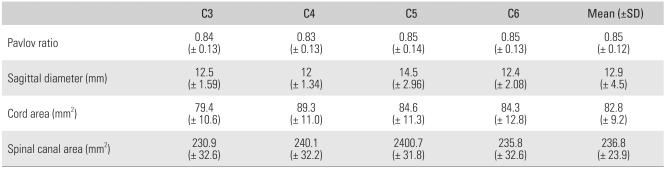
Pavlov Ratio in the Plain X-ray and Sagittal Diameter, Cord Area, Spinal Canal Area in MRI (Mean ± 2 SD)
